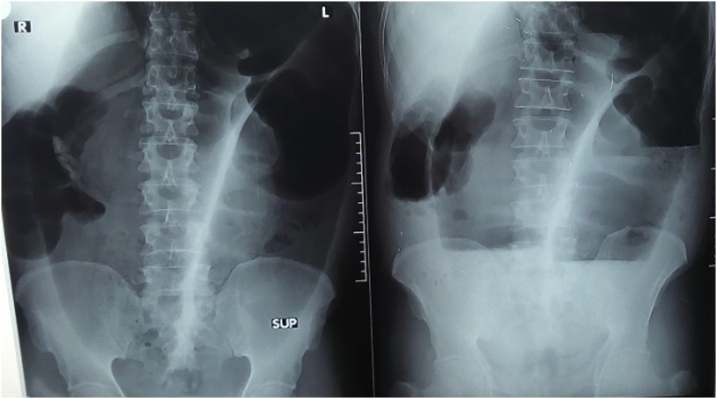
Erect and supine plain abdominal X-rays.
